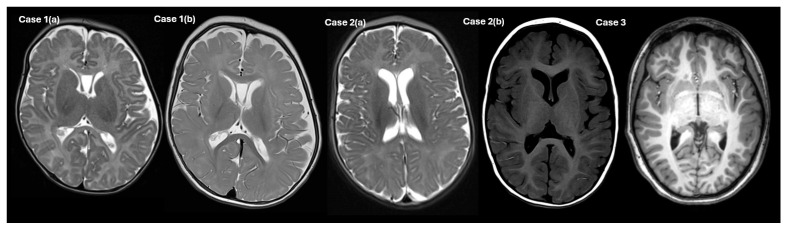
MRI brain images. Comparison of axial images of Case 1 at 3 months (a, T2 axial) and 7 months (b, T2 axial), demonstrating delayed white matter myelination, white matter volume loss and prominent cavum septum pellucidum. Case 2 at 5 months (a, T2 axial) and 20 months (b, T1 axial), showing progression of white matter loss. Case 3’s MRI brain was normal.
